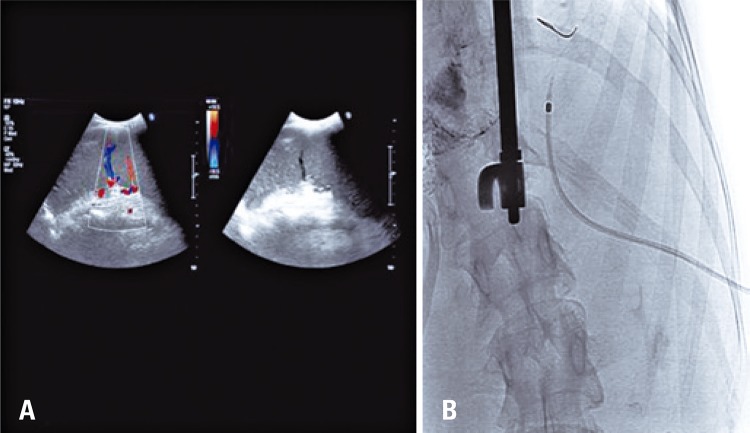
Transsplenic access. (A) Ultrasonography with Doppler of spleen to identify artery and splenic vein, and choice of the best site for puncture. (B) Transsplenic puncture. Kit of micropuncture Neff Percutaneous Assessment Set (NPAS. Cook Medical®)
